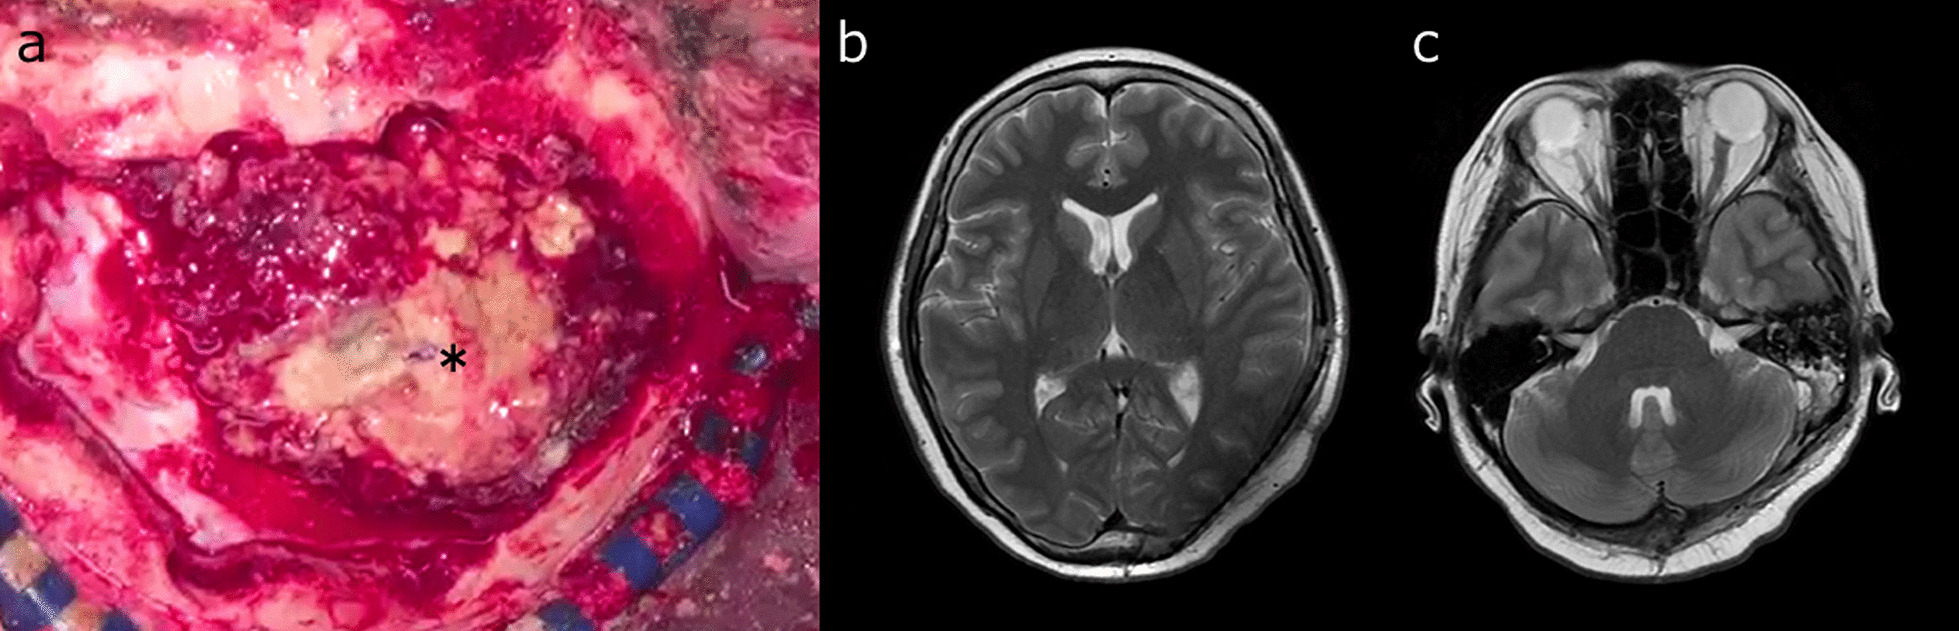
Craniotomy was performed across the transverse sinus. A yellowish-white tumor (asterisk) was observed in the epidural space (a). Head MRI after intensity-modulated radiation therapy shows the residual tumor in the left mastoid air cells and sigmoid sinus; however, it is smaller than that before radiotherapy (b, c)
